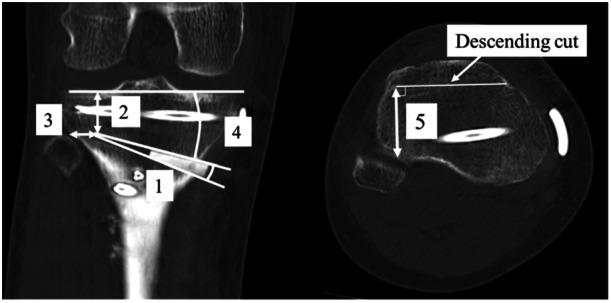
Coronal and axial computed tomography 2 weeks postoperatively. (1) Angle of opening wedge; (2) distance between hinge and the tibiofemoral articular surface; (3) distance between hinge and lateral margin of tibia; (4) angle of osteotomy between the tibiofemoral articular surface and osteotomy line; (5) anterior‐posterior width of hinge.
